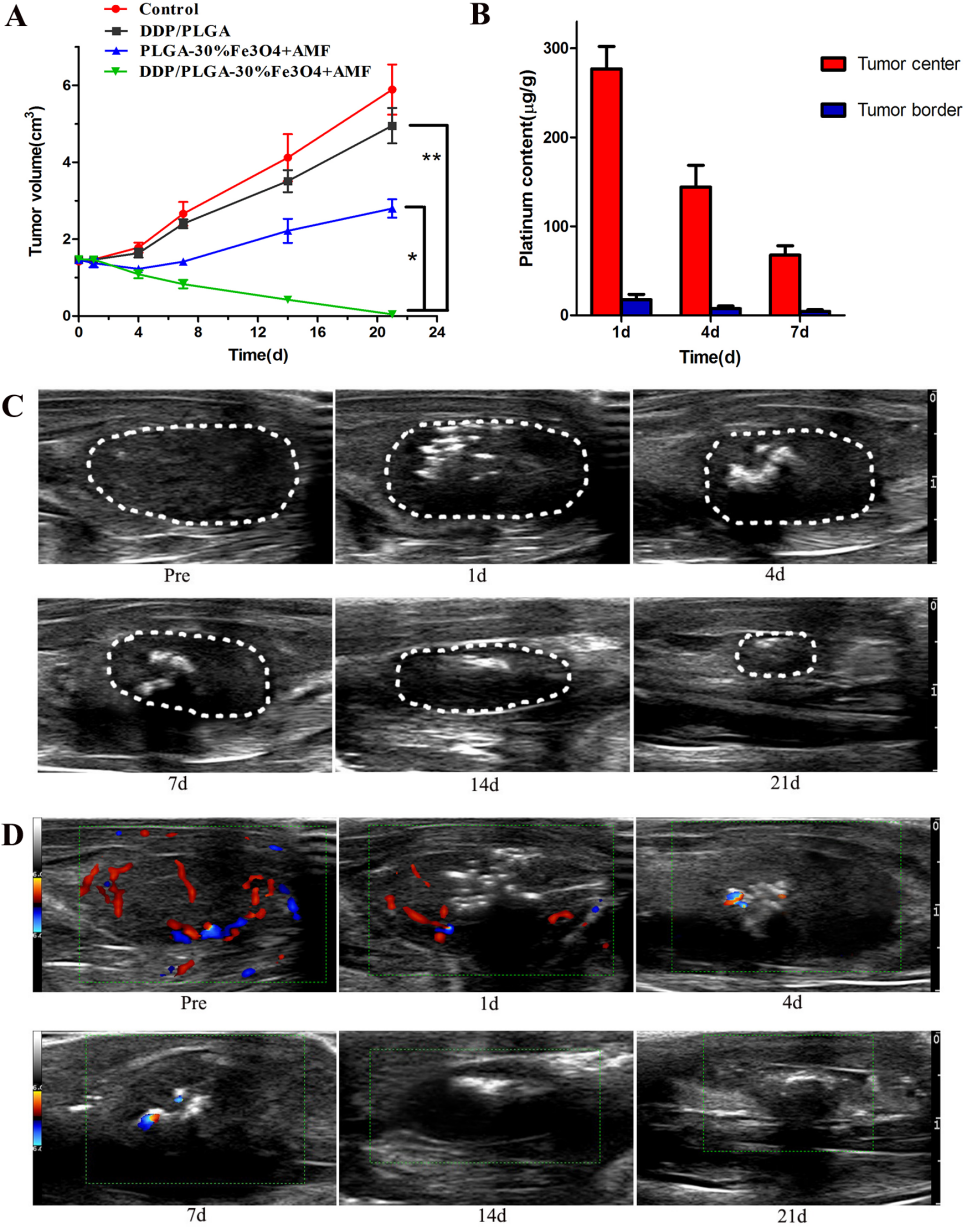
In vivo magnetic thermal therapy and chemotherapy for tumor. (A) The tumor volume–time curve in different groups after ablation (P* < 0.05). (B) Platinum concentration in the center of the tumor and in the edge of the tumor in the DDP/PLGA-30% Fe + AMF group. (C) US 2D images of tumors in the DDP/PLGA-30% Fe + AMF group prior to therapy and on days 1, 4, 7, 14, and 21 after therapy (white circle: the tumor region). (D) CDFI of tumors in the DDP/PLGA-30% Fe + AMF group prior to therapy and on days 1, 4, 7, 14, and 21 after therapy. Behind the hyperechoic implants on the images of days 4 and 7 is the color Doppler twinkling artifact.
